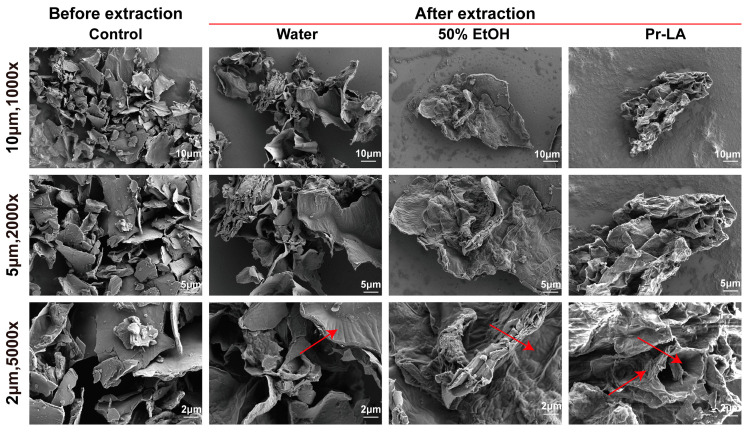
SEM analysis of residues before and after extraction of CLs using ultrasound-assisted combination with different solvents (The red arrow indicates the surface microstructural changes of the powder sample).
